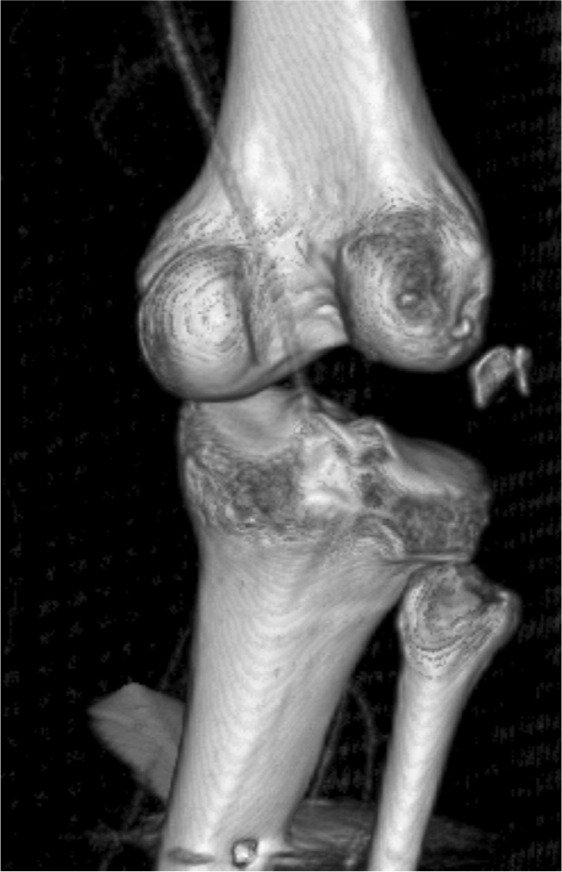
3D reconstruction Computed Tomography (CT Imaging) revealed thrombosed of right popliteal artery on dislocated knee.
